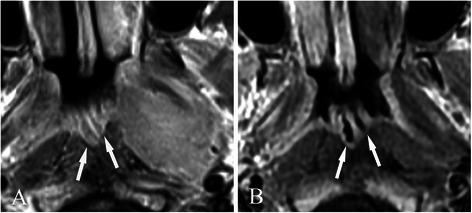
Axial T1-weighted contrast-enhanced MR images of a 16-year-old child who had NPC coexisting with hypertrophic adenoids. A, before treatment, dark stripes are observed in hypertrophic adenoids (arrows). B, 3 months after radiotherapy, the stripes disappear and are replaced by air (arrows).
